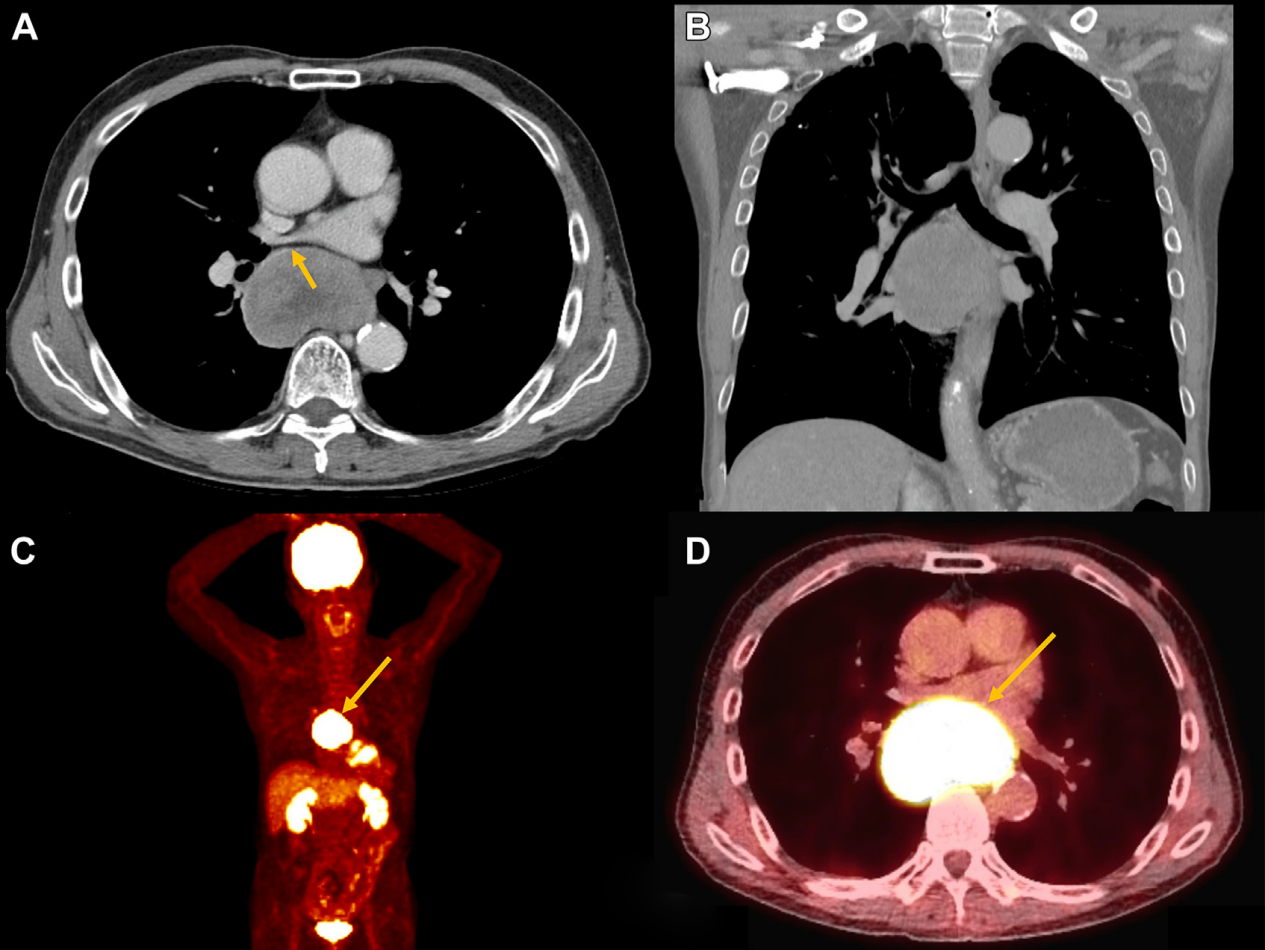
Preoperative computed tomography and fluorodeoxyglucose positron emission tomography findings. (A, B) Heterogeneous subcarinal mass shown abutting the left atrium, right bronchus, and esophagus. (C, D) Fluorodeoxyglucose positron emission tomography scan showing an intensely hypermetabolic subcarinal mass without evidence of distant or regional metastasis. Arrows indicate subcarinal thymic adenocarcinoma.
